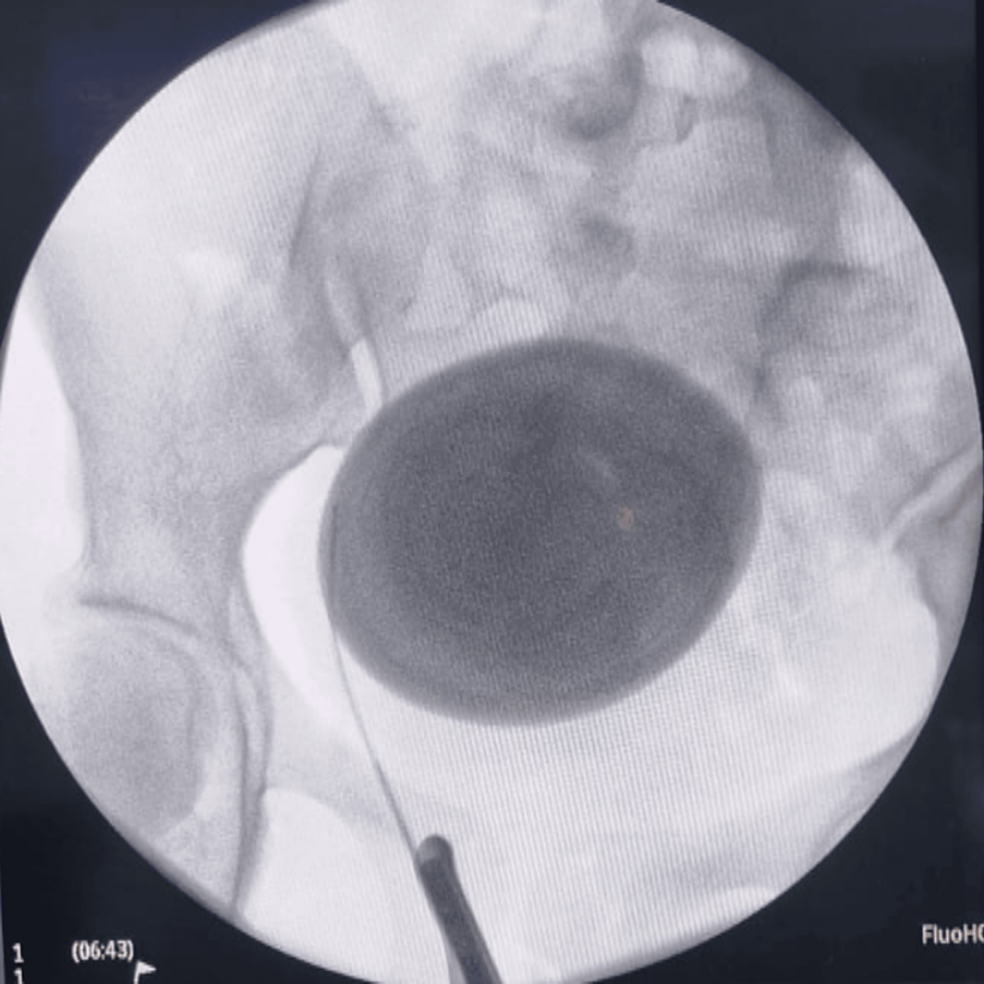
X-ray fluoroscopy with right retrograde pyelogram showing no stone in right ureter
